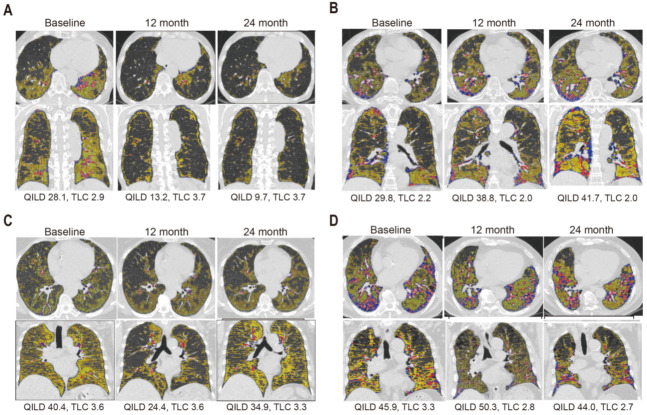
Four cases representing dynamic change of the severity of RA-ILD during 2 years (cohort 2). QILD scores were calculated for each patient who had 3 HRCT scans with 1 year interval without obvious acute exacerbation: (A) Improved case (Patient 9), (B) Worsened (Patient 5), (C) Convex (Patient 12), and (D) Concaved changed (Patient 3). Upper row: transverse view; Lower row: coronal view; QLF = sum of red and blue dot, QGG = yellow dot. Four distinct patterns of change in 3 longitudinally-obtained QILD scores (cohort 2, *n* = 24). (A) Whole lung QILD sc.
